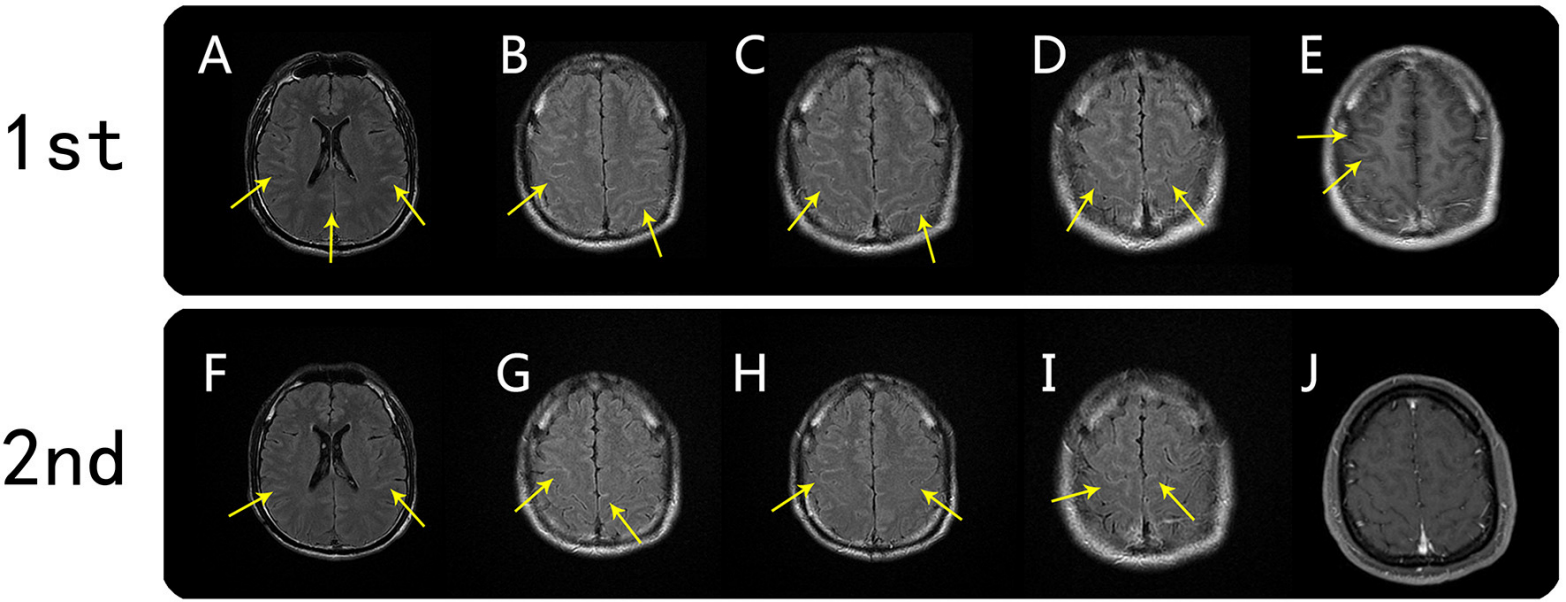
Brain MRI findings from the first and second scans. First MRI scan (on day 2): axial FLAIR hyperintensity was seen in the sulci of the bilateral frontal, temporal, and parietal lobes (A–D, arrows). Corresponding meningo-cortical enhancement was also seen on axial T1-weighted postgadolinium-enhanced images (E, arrow). Second MRI scan (on day 7): meningo-cortical lesions on axial FLAIR were mildly regressed, but abnormalities were still observed (F–I, arrows). T1-weighted postgadolinium-enhanced image showed a corresponding meningo-cortical lesion with no enhancement (J).
